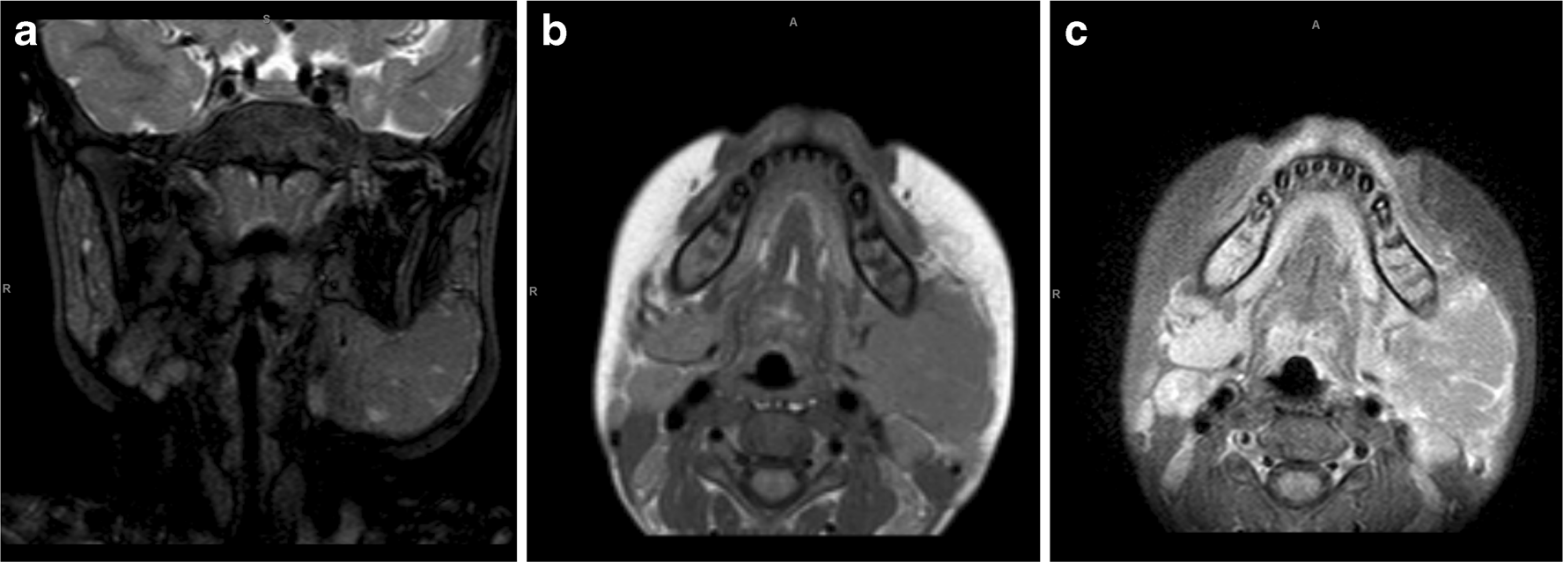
Burkitt lymphoma. Coronal T2-W (a) and T1-W pre-contrast (b) MRI show a subcutaneous mass posterior to the left mandibular angle which is iso-intense on both sequences. On the axial T1-W post-contrast scan (c), there is heterogeneous enhancement and associated posterior triangle lymphadenopathy
